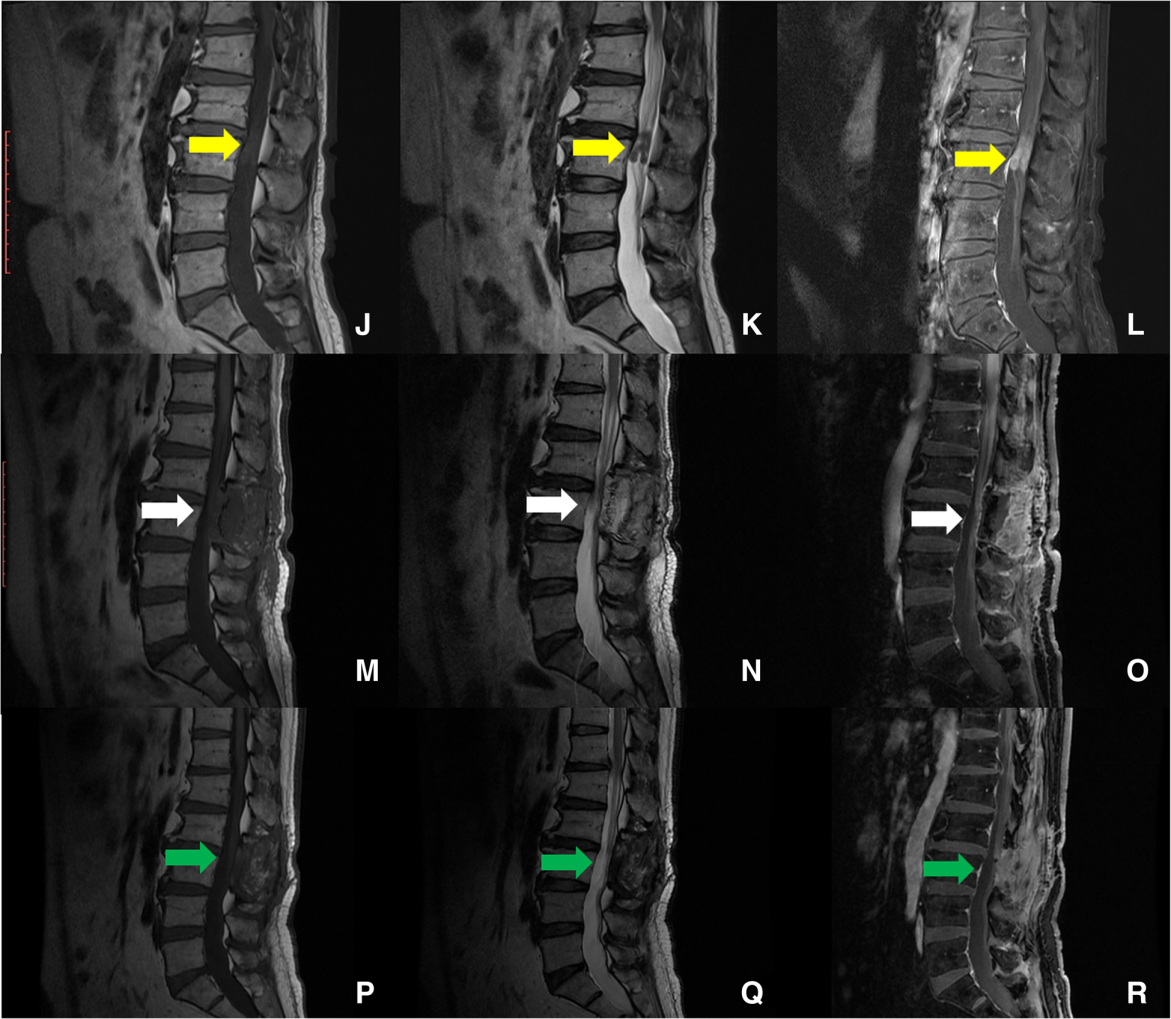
MRI images in sagittal sequence before operation showing isointense on T1WI (j) and hypointense on T2WI(k), with remarkable homogenous enhancement(l). 4 days (m,n,o)and 9 months(p,q,r) post-operation MRI showing totally resection of the tumors in corresponding sequences. The yellow arrows point to the tumor, the white and green arrows showed totally tumor resection with disappearance of enhancement
